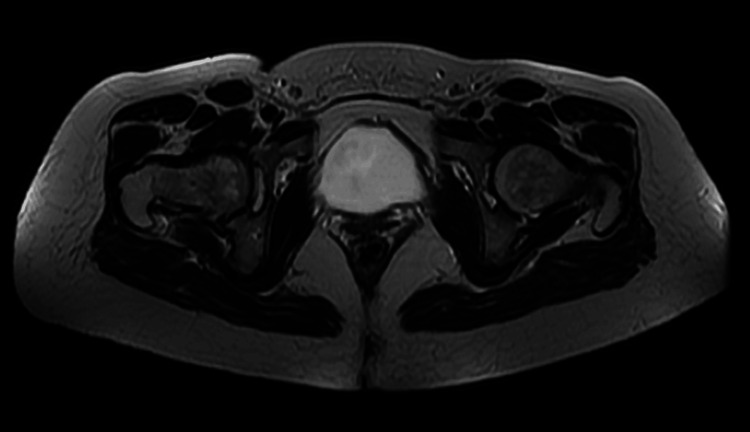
Pelvic and upper right leg low-resolution MRI scan (T2) performed four months after hospitalization. At this point, it was only visible a residual T2 hyperintensity in the deeper region of the right buttock, with no visible collections.
